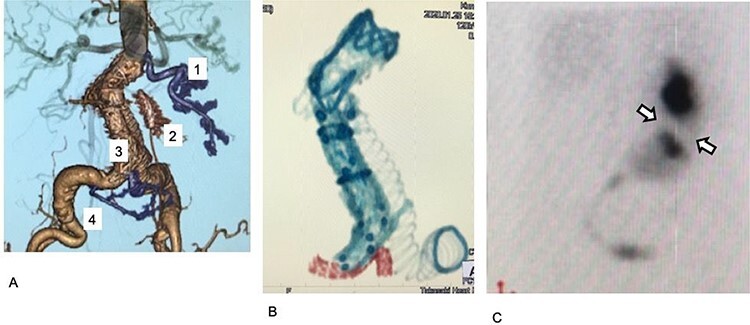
Postoperative images. (A) Postoperative enhanced CT. The first, third, and fourth RA are preserved (purple). The third renal artery is preserved by a chimney graft with good perfusion. (B) Stent grafts and the covered stent on postoperative plane CT. The covered stent is shown in red. The stent tracks well to the acute angle of the third renal artery. (C) Postoperative 99mTc-MAG3 renogram. Collection ceased in a small region in the kidney, which was assumed to be perfused by the second renal artery (arrow).
